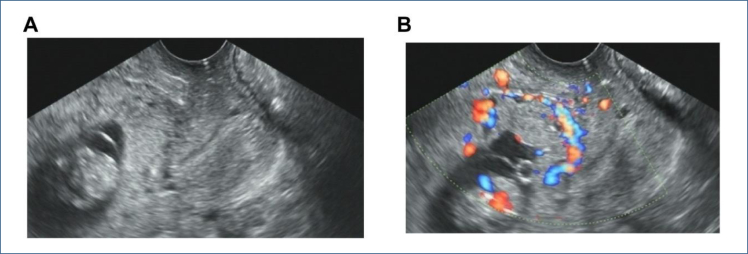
Transvaginal ultrasound image of a patient at 10 weeks and 3 days gestation with type III cesarean scar pregnancy. The ultrasound reveals that the placenta entirely overlies the internal cervical os, with the posterior edge of the placenta being entirely overlies the internal cervical os, with the posterior edge of the placenta being indistinguishable from the lower segment of the anterior uterine wall myometrium. There appears to be a discontinuity in the serosal layer (A). Color Doppler flow imaging demonstrates abundant blood flow signals localized to the scar site (B).
